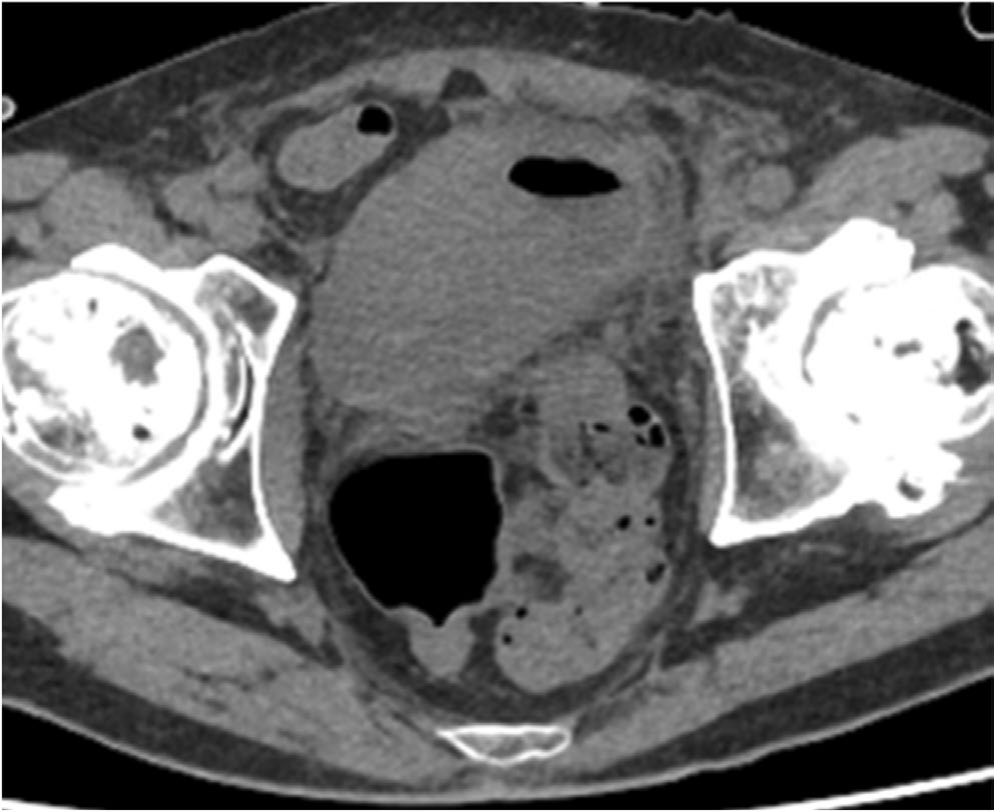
The chest and abdominal CT findings reveal a significant reduction in the size of the bladder tumor.
